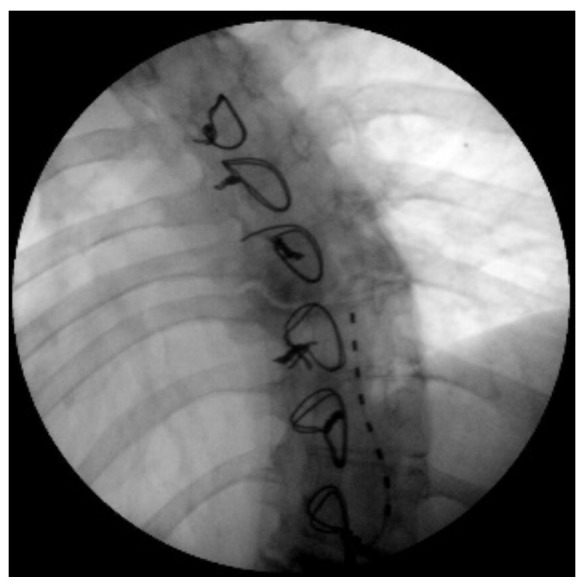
X-ray image of the subcutaneous catheter positioned between D8 and D10.
